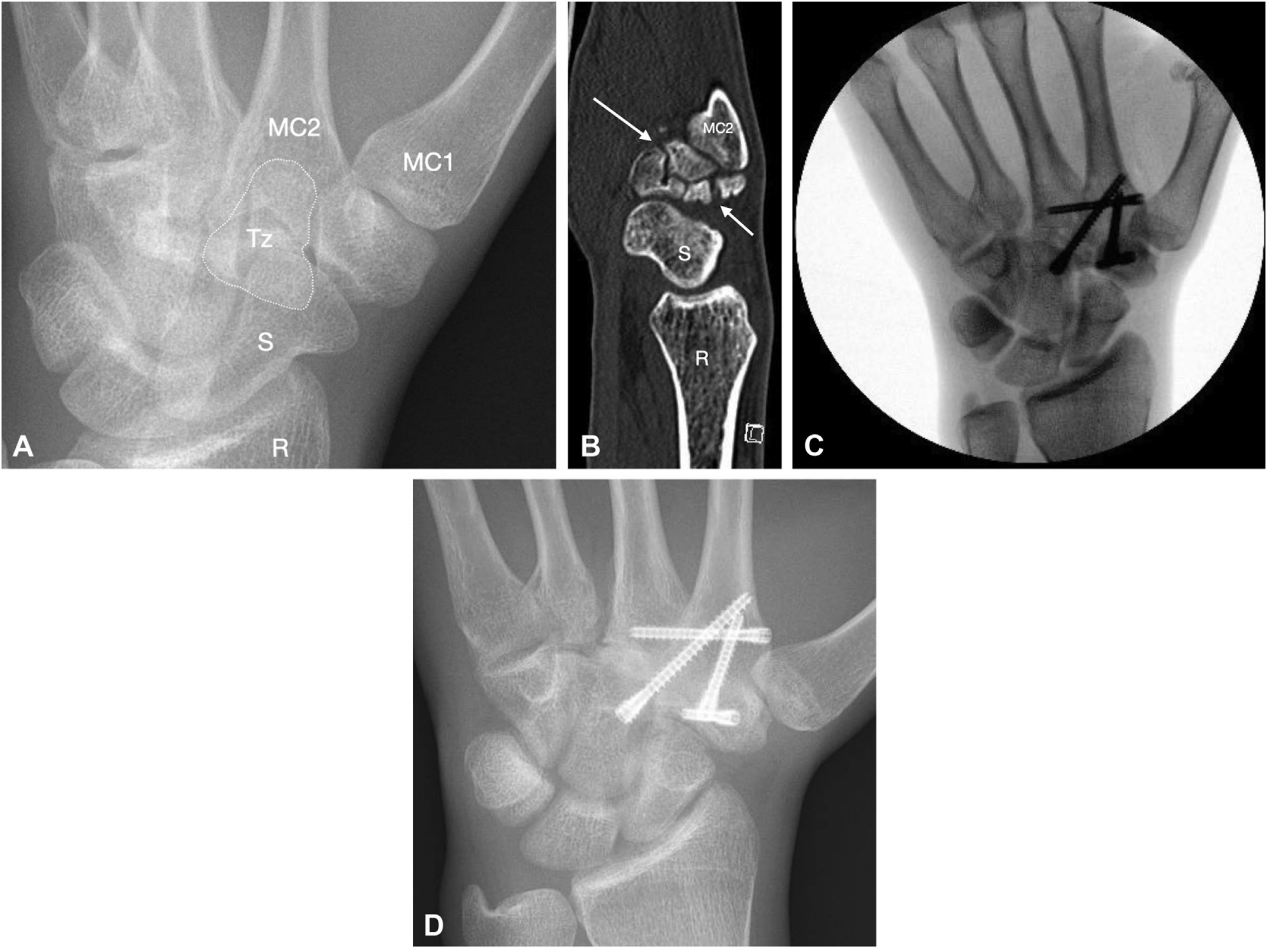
Images of the injury reported in case 2. A x-ray antero-posterior (AP) view view that was initially interpreted as normal, but closer inspection shows an abnormal appearance of the trapezoid (dotted line). Tz = Trapezoid; MC1 = metacarpal 1; MC2 = metacarpal 2; S = scaphoid; R = radius. B CT scan sagittal view showing a markedly displaced and comminuted trapezoid fracture nonunion (arrows). MC2 = metacarpal 2; S = scaphoid; R = radius. C Intraoperative fluoroscopy image after completed carpometacarpal (CMC) II and III arthrodesis with screw fixation and bone graft. D Six weeks postoperative X-ray AP view showing healed 2nd and 3rd carpometacarpal arthrodesis with screws in situ.
